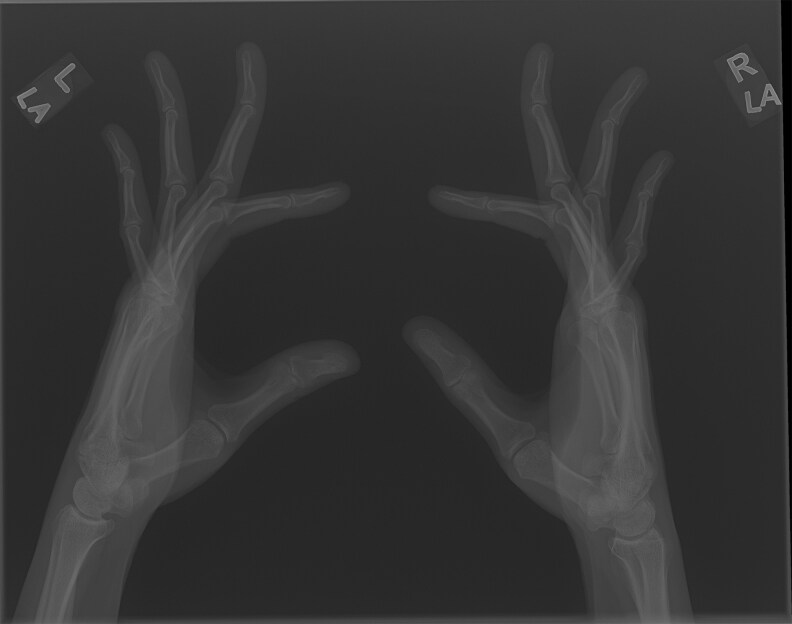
Arachnodactyly of hands on x-ray.
